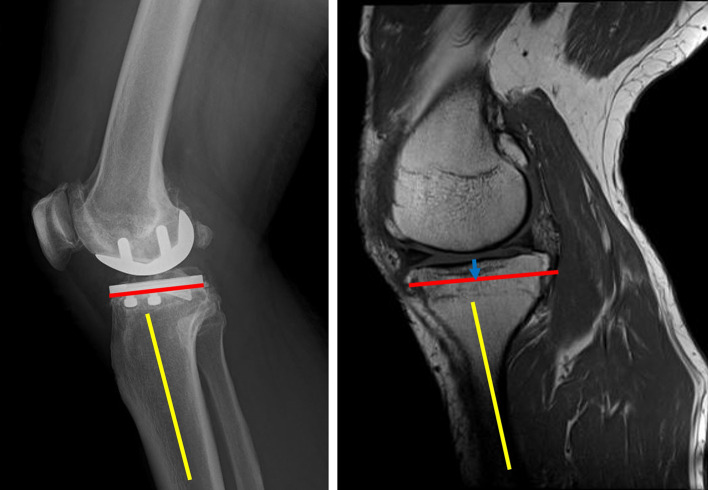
The tibial posterior tilt angle was measured from the postoperative lateral radiograph image. The amount of tibial resection was determined from the surgical record (blue arrow), and a line was drawn parallel to the tibial posterior tilt angle on the sagittal MRI image to create a virtual tibial cut line (red line).
